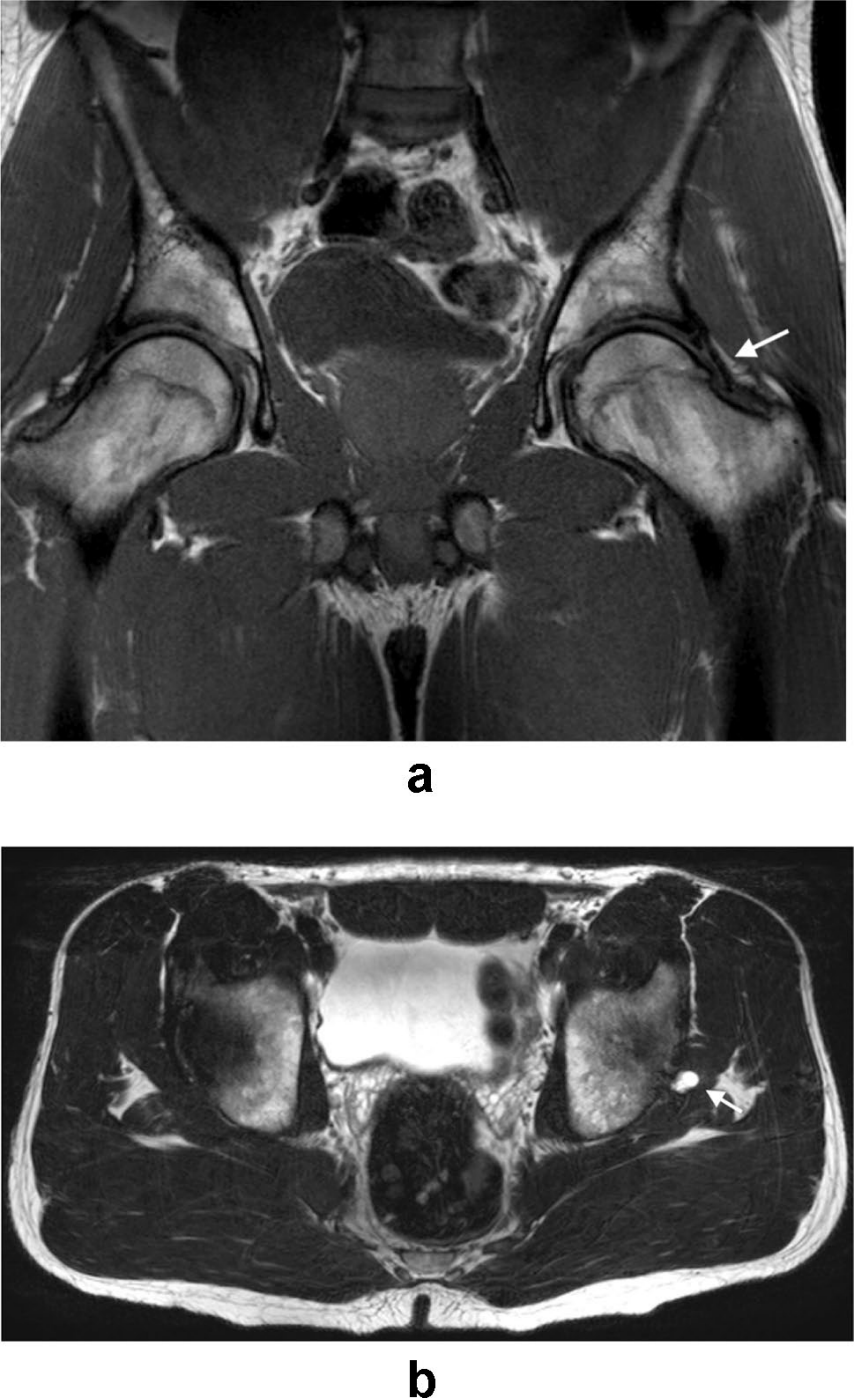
A Coronal T1-weighted image of the pelvis in a 34-year-old male athlete demonstrating subtle protuberance at the femoral head-neck junction (arrow) in keeping with Cam-type impingement morphology. There is associated early degenerative partial-thickness chondrosis at the hip joints. B Axial T2-weighted image in the same patient demonstrating a small left paralabral cyst (arrow) consistent with an underlying labral tear
